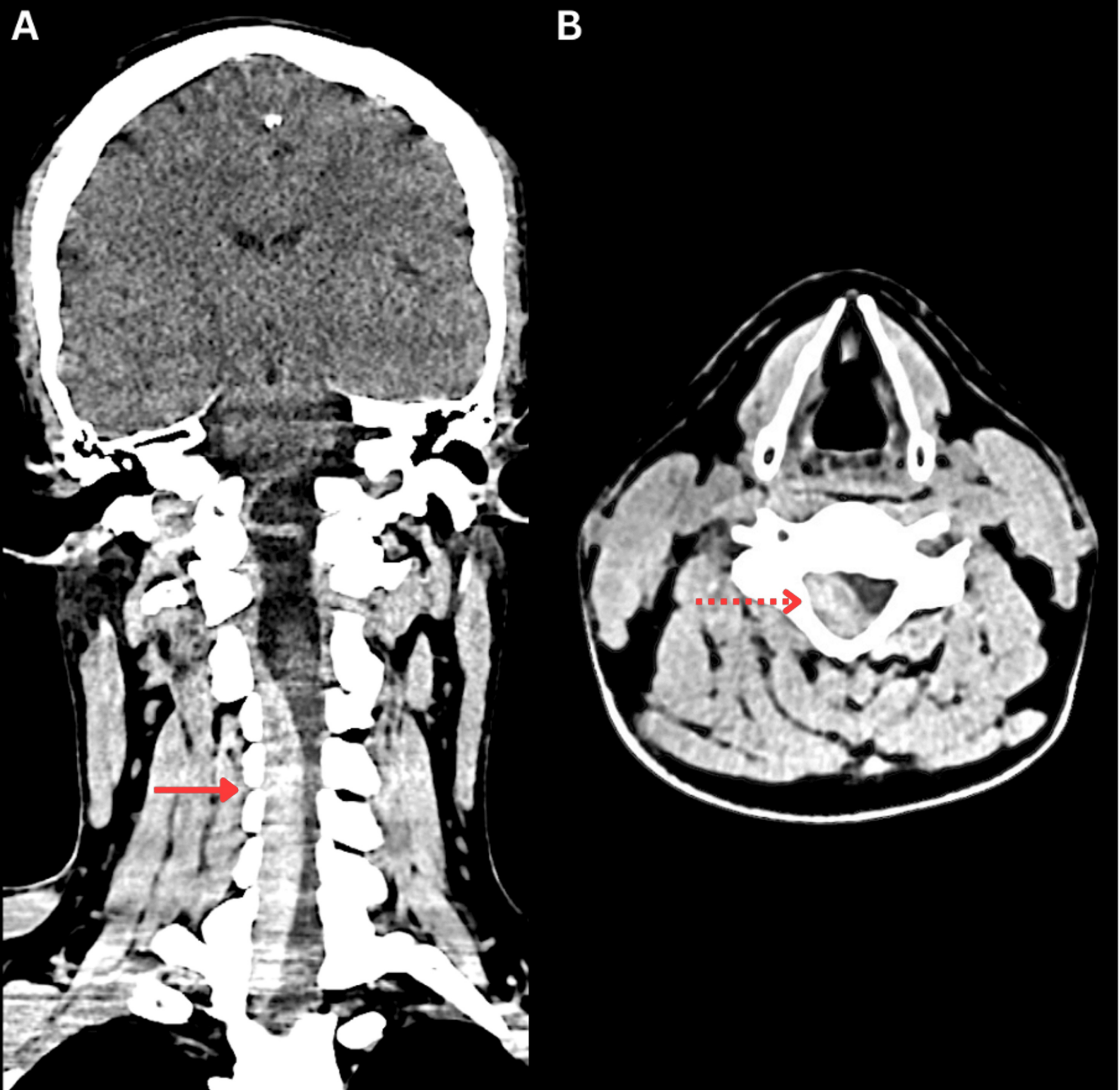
Non-contrast brain and cervicothoracic CT scan showing an epidural hematoma causing spinal cord compression. (A) Coronal plane: hyperdense epidural collection extending from C3 to T2 (solid red arrow). (B) Axial plane at C6: anterior displacement of the spinal cord (dashed red arrow).
